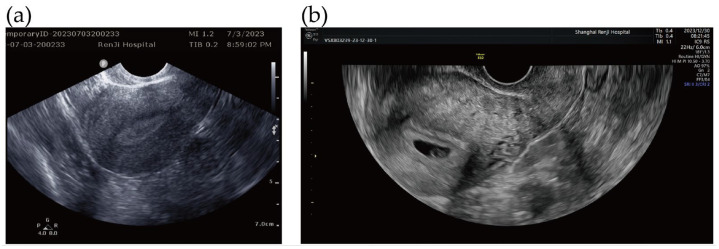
(a) Follow-up ultrasound obtained three months after hysteroscopic resection showed no residual mass and a well-healed uterine cavity. (b) Early pregnancy transvaginal ultrasound confirmed a viable intrauterine pregnancy after eight months of the surgery.
